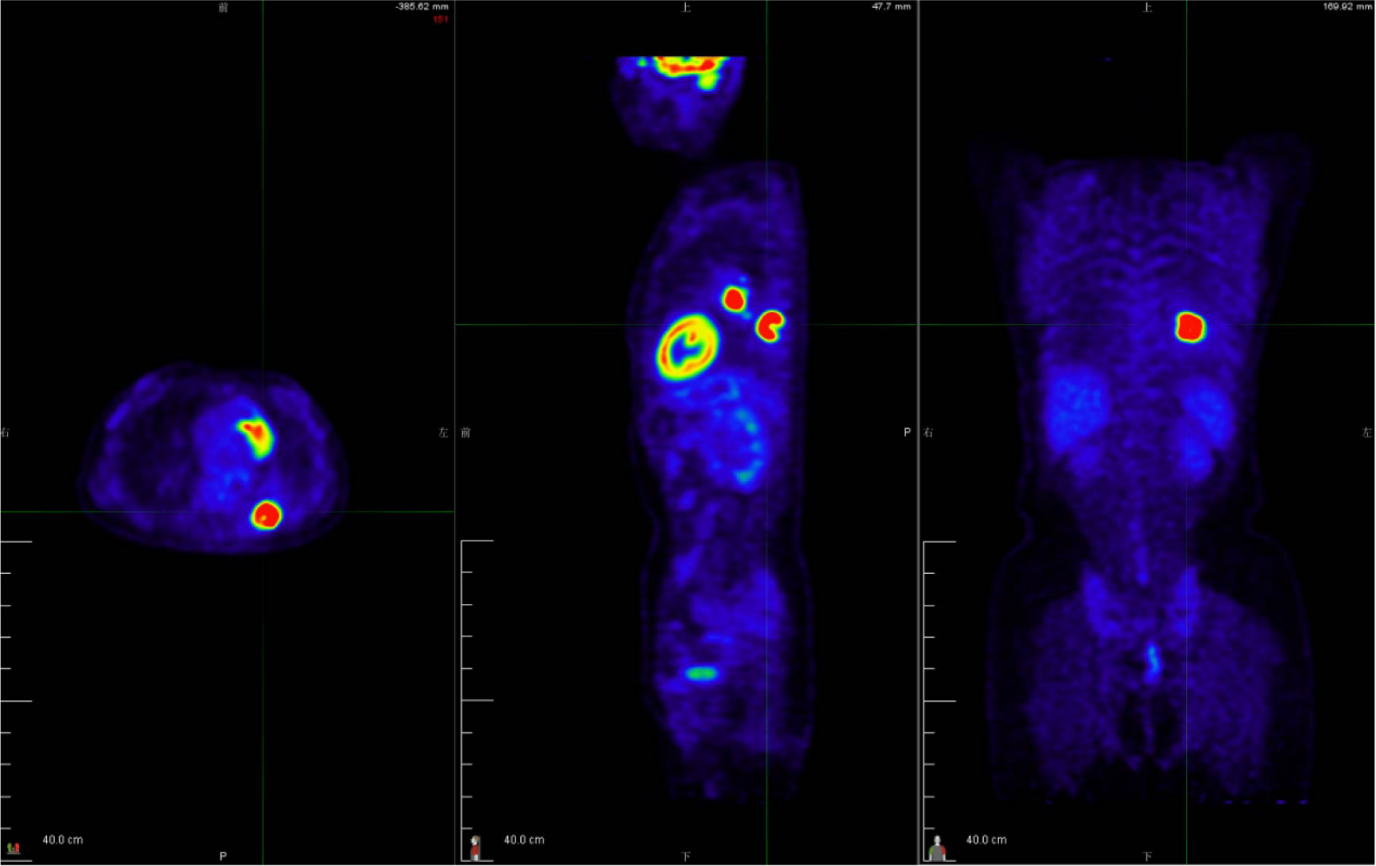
The 18F-FDG PET/CT examination was performed to evaluate the patient’s tumor stage. The 18-FDG PET/CT revealed strong 18-FDG uptake in the left lung mass, as well as the left hilar and subcarinal lymph node, measuring up to 2.5 cm and 2.2 cm in short diameters, respectively, but there was no evidence of distant metastasis. See Supplementary Material for a dynamic 3D visualization.
